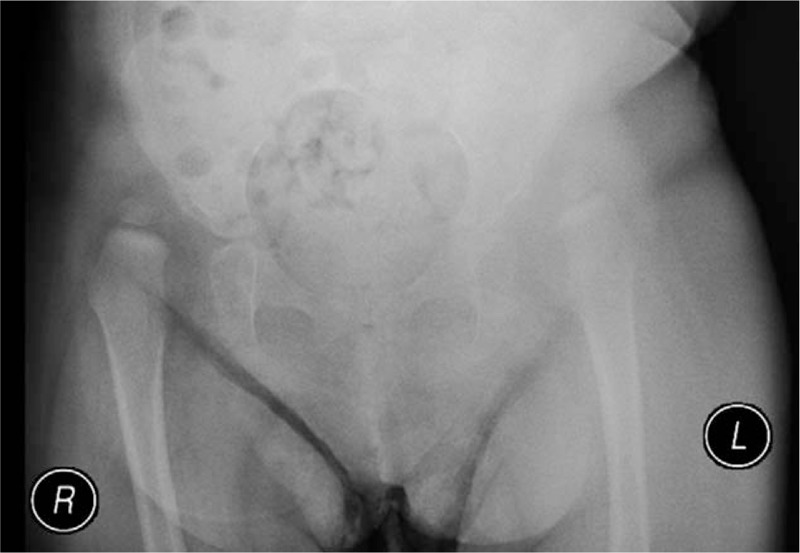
Fourteen-month-old girl with bilateral developmental dysplasia of the hips: Anteroposterior radiograph of the pelvis showing dislocation of both hips.
